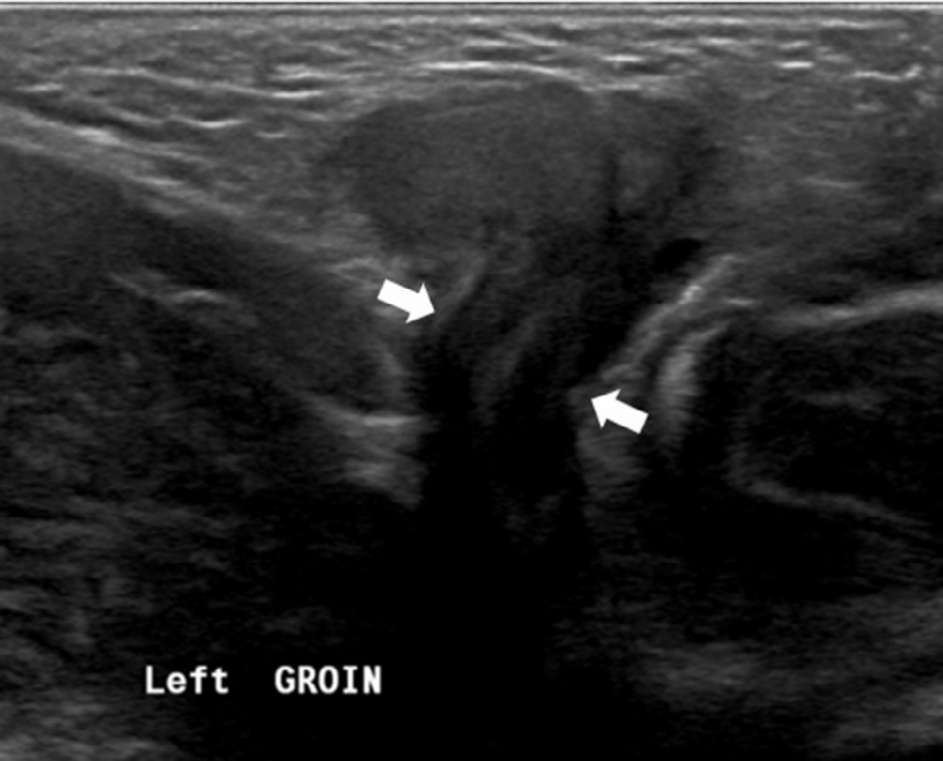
Grayscale ultrasound image showed a defect in the left inguinal area with a herniated uterus.
